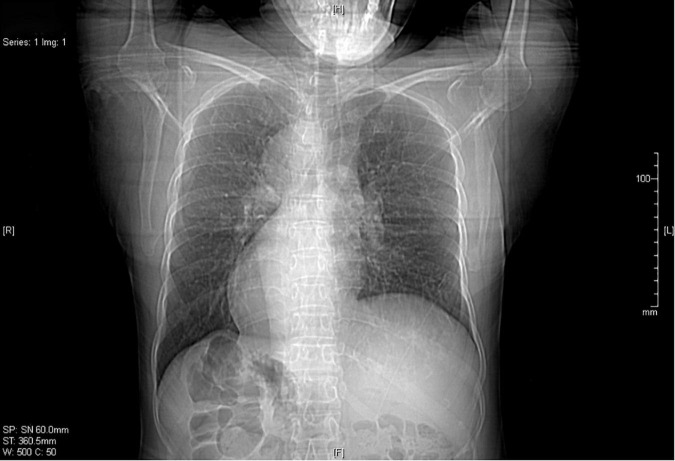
Chest X-ray shows dextrocardia in this patient with situs inversus.
